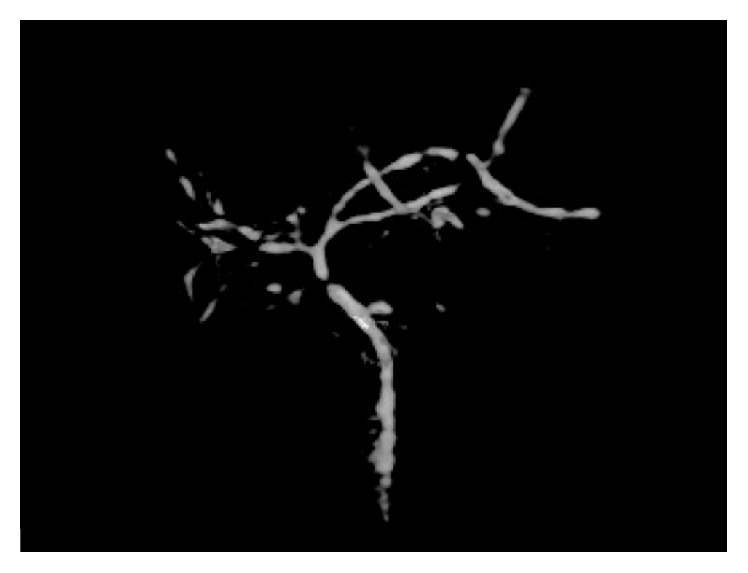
3D MRI cholangiogram shows intrahepatic and extrahepatic biliary duct irregularity, with choledochus stenosis.
